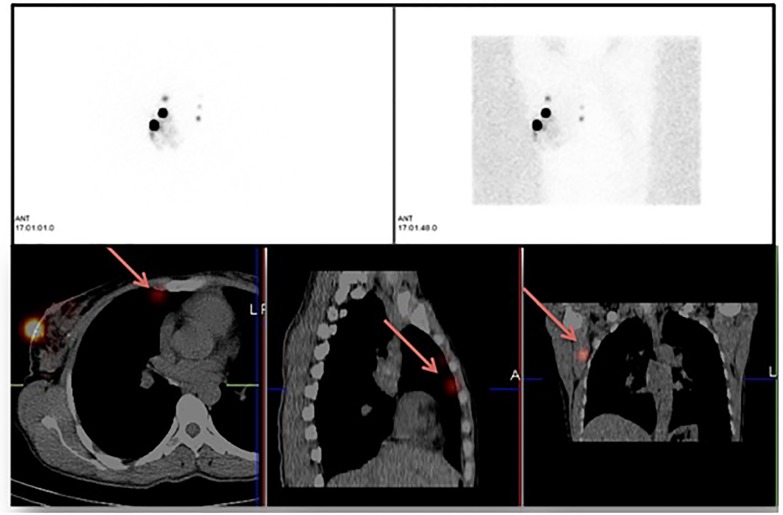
PS images in a female with right breast cancer showed multiple uptakes in medial quadrants of right breast in addition to injection sites. SPECT/CT images localized tracer uptake in right level I axillary SLN and multiple internal mammary chain nodes
